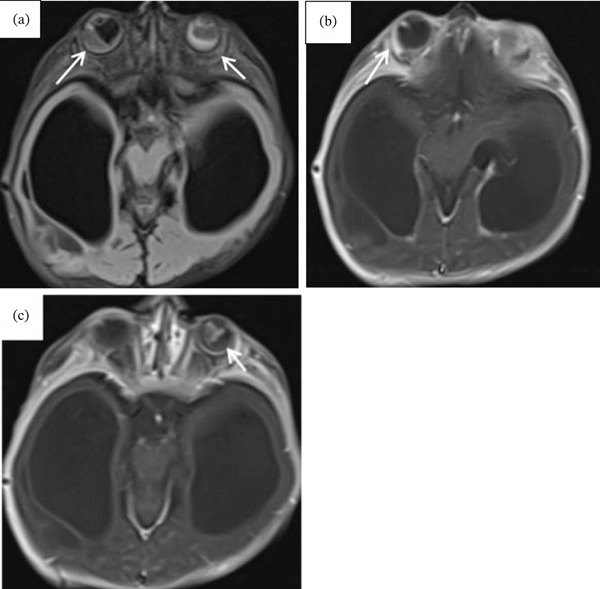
Bilateral chorioretinitis. (a) Axial FLAIR MRI demonstrates hyperintense signal involving the bilateral chorioretina. (b, c) Postcontrast fat‐suppressed axial T1‐weighted images show corresponding enhancing lesions.
